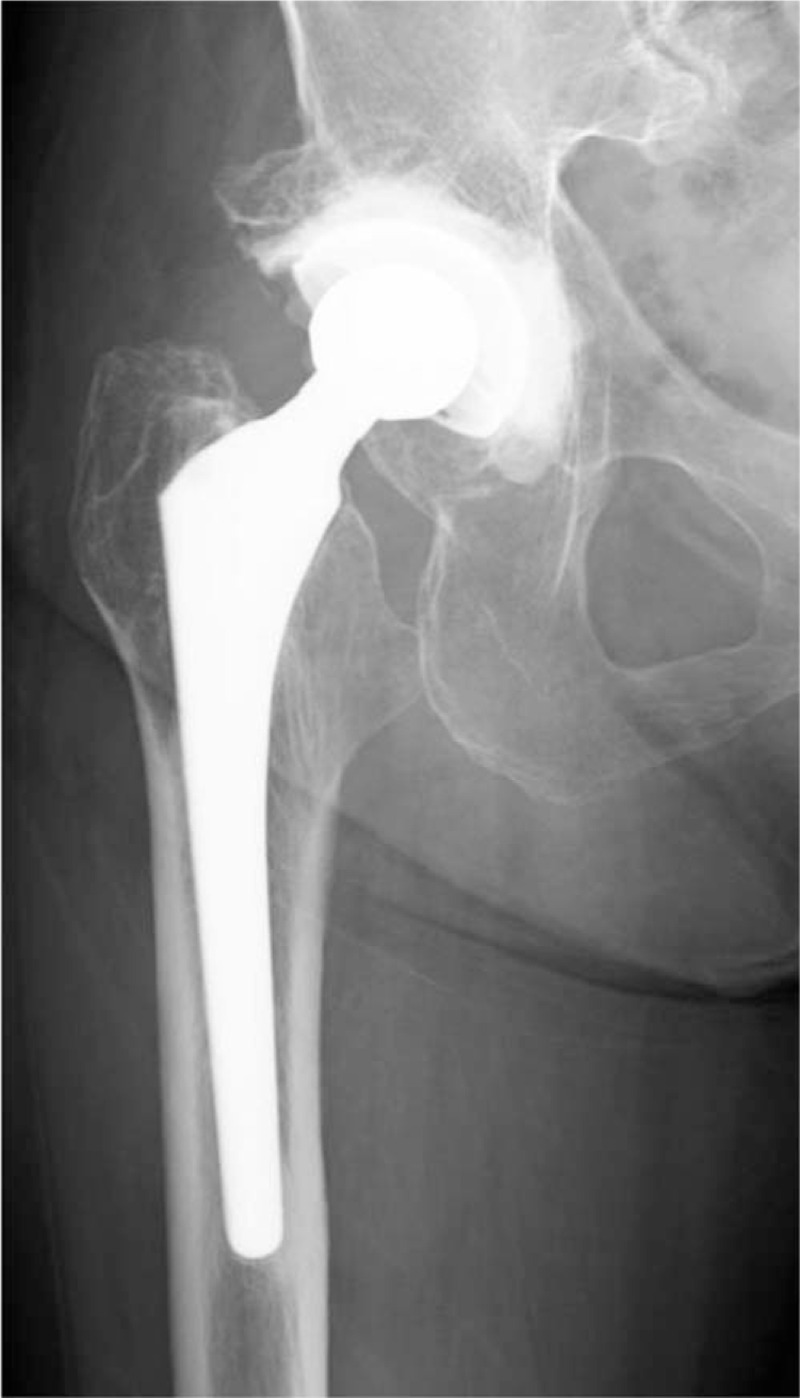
Plain radiograph immediately after surgery. The implant is retained, the wire and screw were removed.
